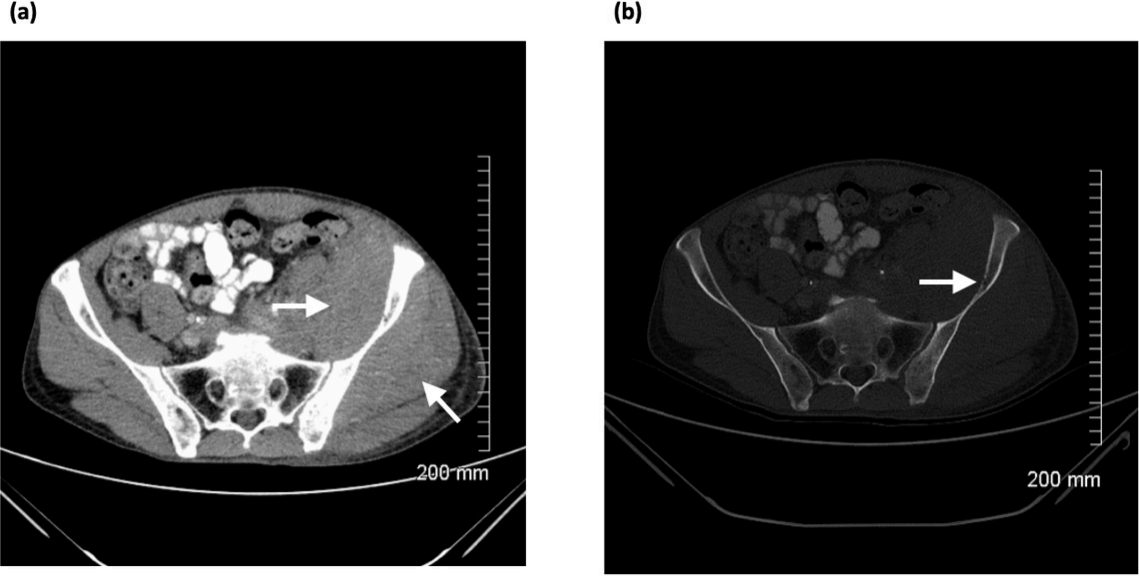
(a) Axial CT image showing large soft tissue mass infiltrating the left iliopsoas and gluteal muscle which is isodense to adjacent musculature, (b) axial CT image of same lesion with bony window demonstrating subtle permeative destruction of the left iliac bone.
